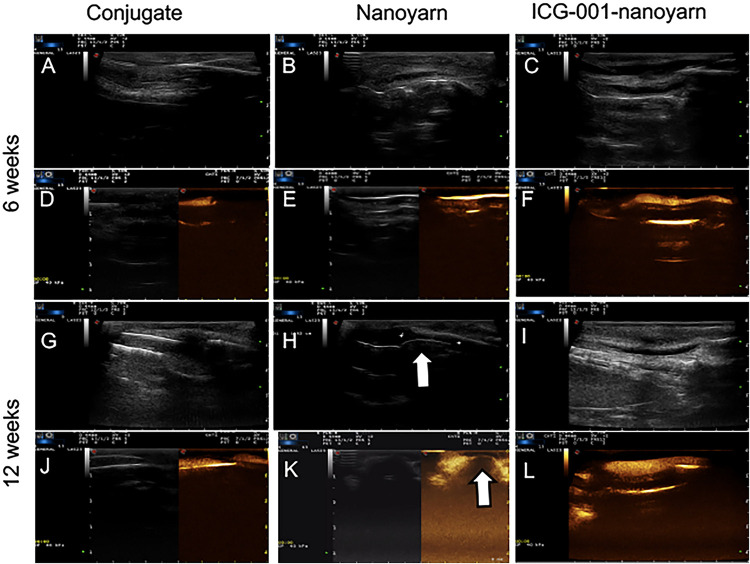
Sonourethrography and ultrasonic contrast examinations for the urethras repaired with conjugated nanofibrous scaffold, nanoyarn and ICG-001-delivering nanoyarn. (A–C,G–I) Sonourethrography examination results. (D–F,J–L) Ultrasonic contrast examination results. The white arrows indicated the location of scar formation in the urethral lumen.
